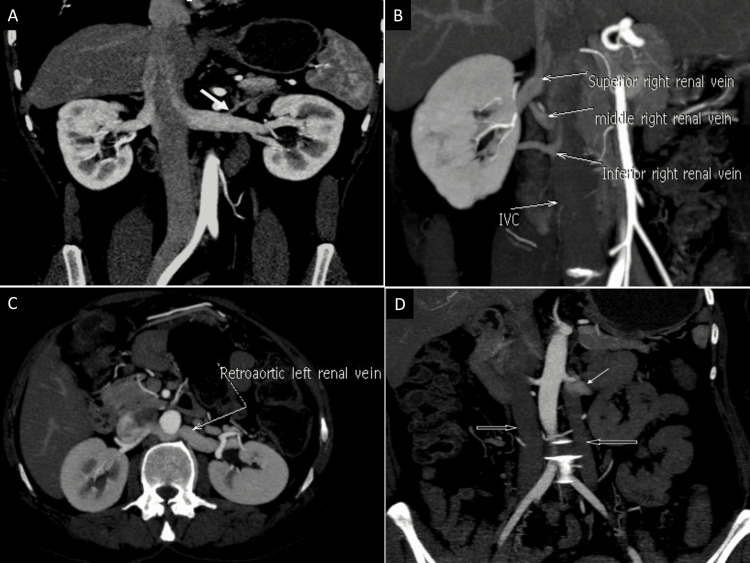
Computed tomography angiographic depiction of renal venous anatomy (A) Post-processing curved coronal reformatted image showing bilateral single renal vein with left adrenal vein draining in to left renal vein (→)  (B) Coronal oblique maximum intensity projection image displaying multiple right renal veins (C) Coronal oblique maximum intensity projection image showing retro aortic left renal vein (D) Coronal maximum intensity projection image displaying double inferior vena cava (⇒) with left inferior vena cava joining left renal vein (→).
